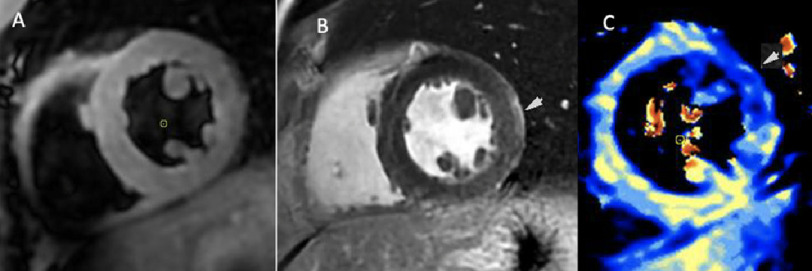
CMR views. A) T2-weight short-tau inversion recovery (STIR) left ventricular mid short axis (SAX) image shows increased signal intensity of the myocardium due to edema. B) Late gadolinium enhancement (LGE) SAX view shows linear subepicardial enhancement in the mid anterolateral segment. C) T2-weighted map shows globally increased T2-values (blue), predominantly in the lateral wall, indicative of myocardial edema.
